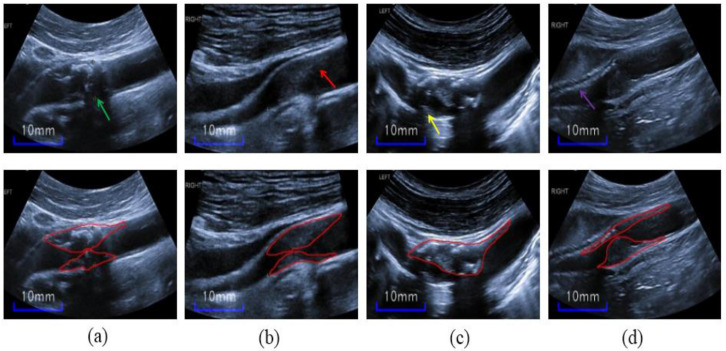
Carotid plaques in ultrasound images from four patients. Top row: (a) The green arrow indicates acoustic shadow, (b) the red arrow indicates speckle noise, (c) the yellow arrow indicates artifacts, and (d) the purple arrow indicates the ambiguous boundary between the plaques and IMC. Bottom row: The red outlines superimposed on the images denote the plaques contours.
